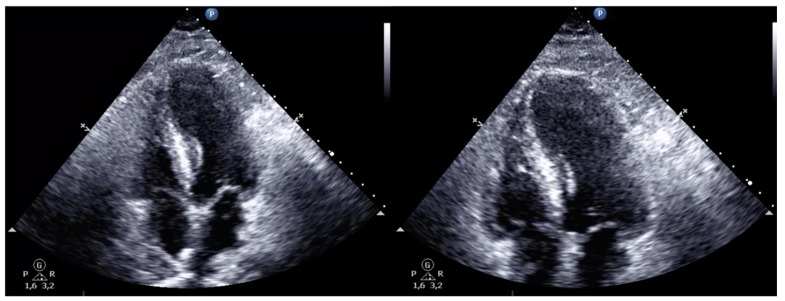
Post-procedure transthoracic echocardiogram (apical 4-chamber view) with preserved biventricular systolic function and normal-sized cardiac chambers.
